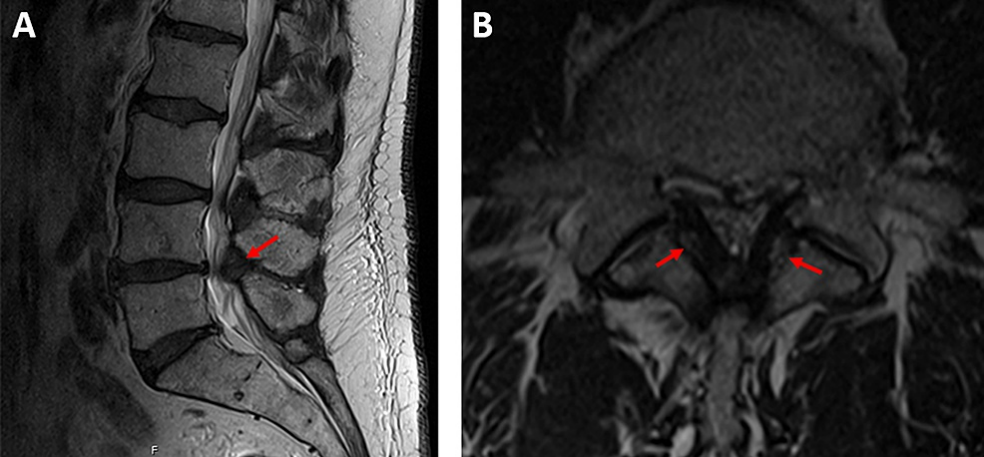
T2-weighted magnetic resonance images (A) Mid-sagittal view depicting severe L4-5 stenosis and ossification of the ligamentum flavum (red arrows). (B) Transverse view depicting severe L4-5 stenosis and ossification of the ligamentum flavum (red arrows).
